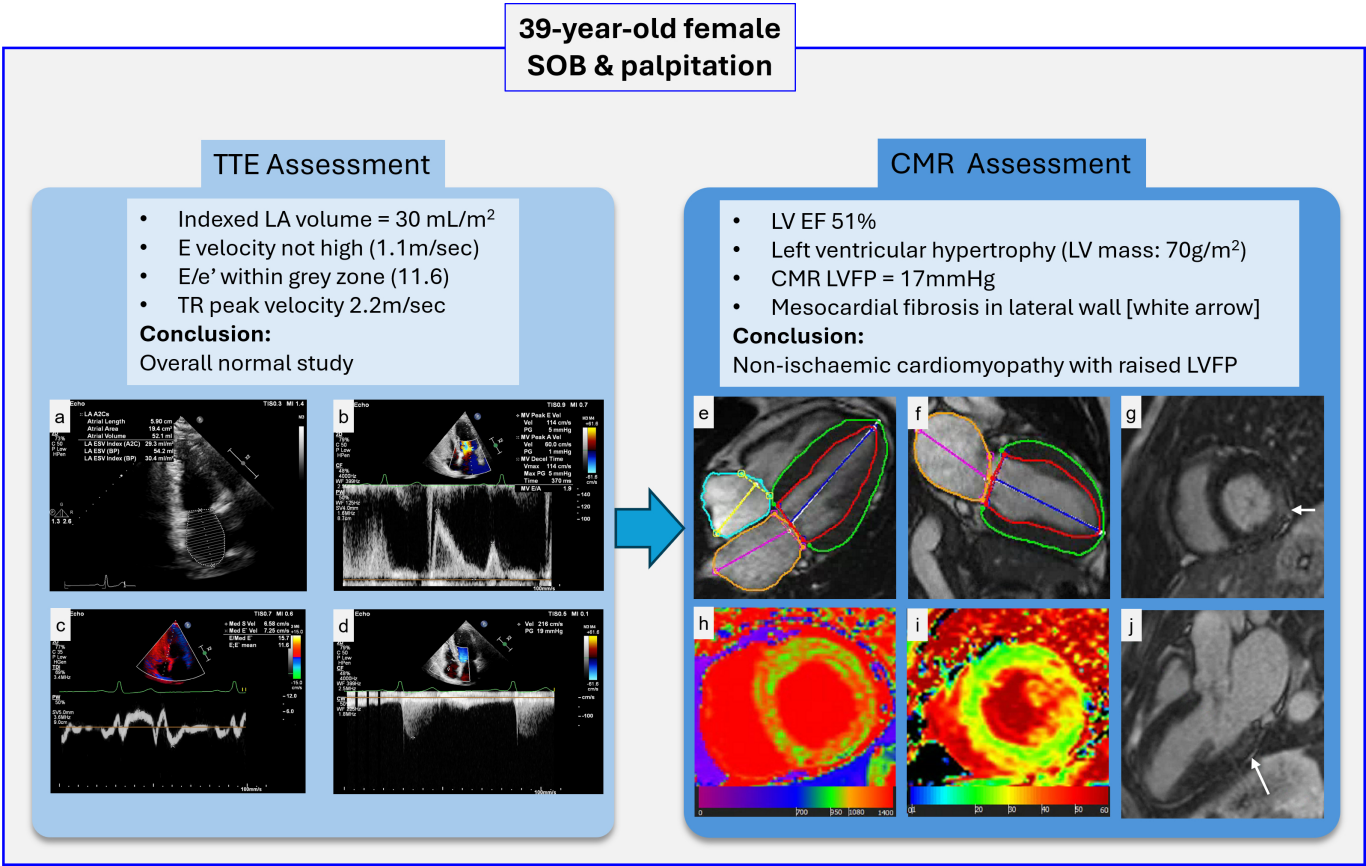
Multimodal cardiac assessment of a 39-year-old female with SOB and palpitations. (a–d) TTE shows an indexed LA volume of 30 mL/m², E velocity of 1.1 m/sec, E/e’ of 11.6, and TR peak velocity of 2.2 m/sec, indicating an overall normal study. (e–g) CMR cine images demonstrate LVH (LV mass: 70 g/m²) with preserved LVEF (51%). (h–i) Native T1 reveals islets of global changes in the myocardium, whereas ECV mapping reveals more focal fibrosis in lateral wall. (g, j) LGE imaging highlights mesocardial fibrosis in the lateral wall (white arrows), consistent with NICM. CMR, cardiac magnetic resonance; ECV, extracellular volume; LA, left atrium; LV, left ventricle; LVEF, left ventricular ejection fraction; LVH, left ventricular hypertrophy; LVFP, left ventricular filling pressure; LGE, late gadolinium enhancement; NICM, non-ischaemic cardiomyopathy; SOB, shortness of breath; TTE, transthoracic echocardiography; TR, tricuspid regurgitation.
